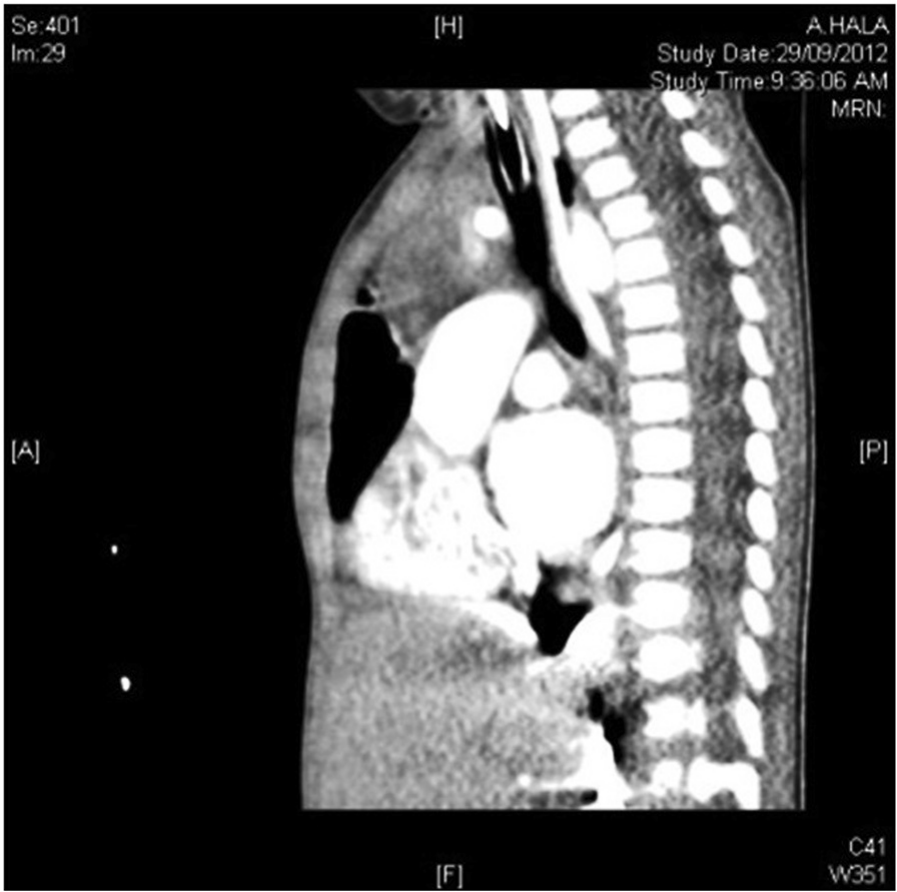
Computed tomography angiography sagittal view shows right aberrant subclavian artery posterior compression to the esophagus at level of T4 vertebra and minimal compression in the posterior trachea
